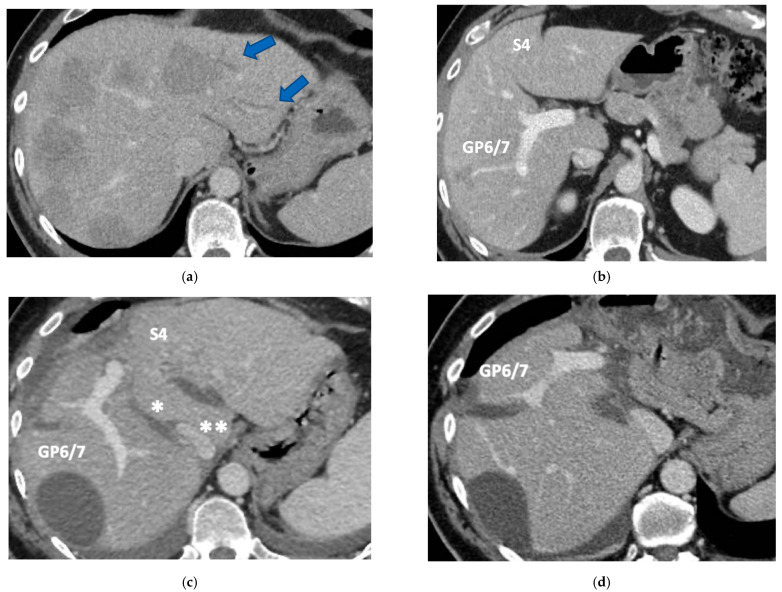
CT Images of PS e-ALPPS performed to treat 19 bilateral CRLMs infiltrating the left first-order glissonean pedicle; FLR is represented by a part of S6 and S7. (a) CT at the diagnosis: arrows indicate biliary dilation due to infiltration of left first-order glissonean pedicle. (b) Partial response after FOLFIRI + Cetuximab 7 cycles; FLR (=part of S6/S7) volume is 17%; segment 4 (S4) and posterior glissonean pedicle (GP 6/7) are indicated. (c) Forty-one days after the first stage FLR (=part of S6/S7) volume increases up to 41%; the first stage of e-ALPPS consisted in resection of S5/S8/caudate processus partially extended to S6/S7 with metastasectomy of S7; this case of e-ALPPS is defined as parenchyma sparing since MHV and S4 were preserved in the deportalized liver (S1-S2-S3-S4); * paracaval portion of S1;  Spiegel lobe. (d) Four and a half months after the PS e-ALPPS the patient is disease free. Abbreviations: PS: parenchyma sparing; e-ALPPS: enhanced ALPPS; CRLM: colorectal liver metastases; FLR: future liver remnant; S: segment GP 6/7: posterior glissonean pedicle for S6/S7; MHV: middle hepatic vein; * paracaval portion of S1;  Spiegel lobe.
